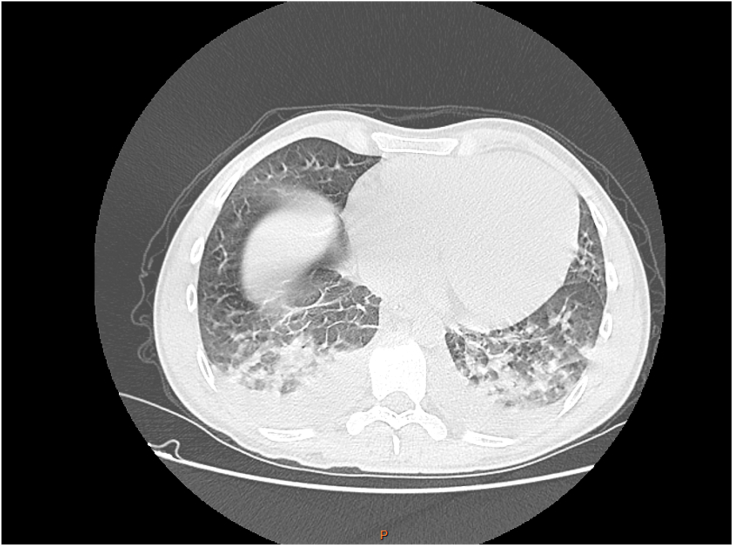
chest computed tomography showing ground-glass opacification.
